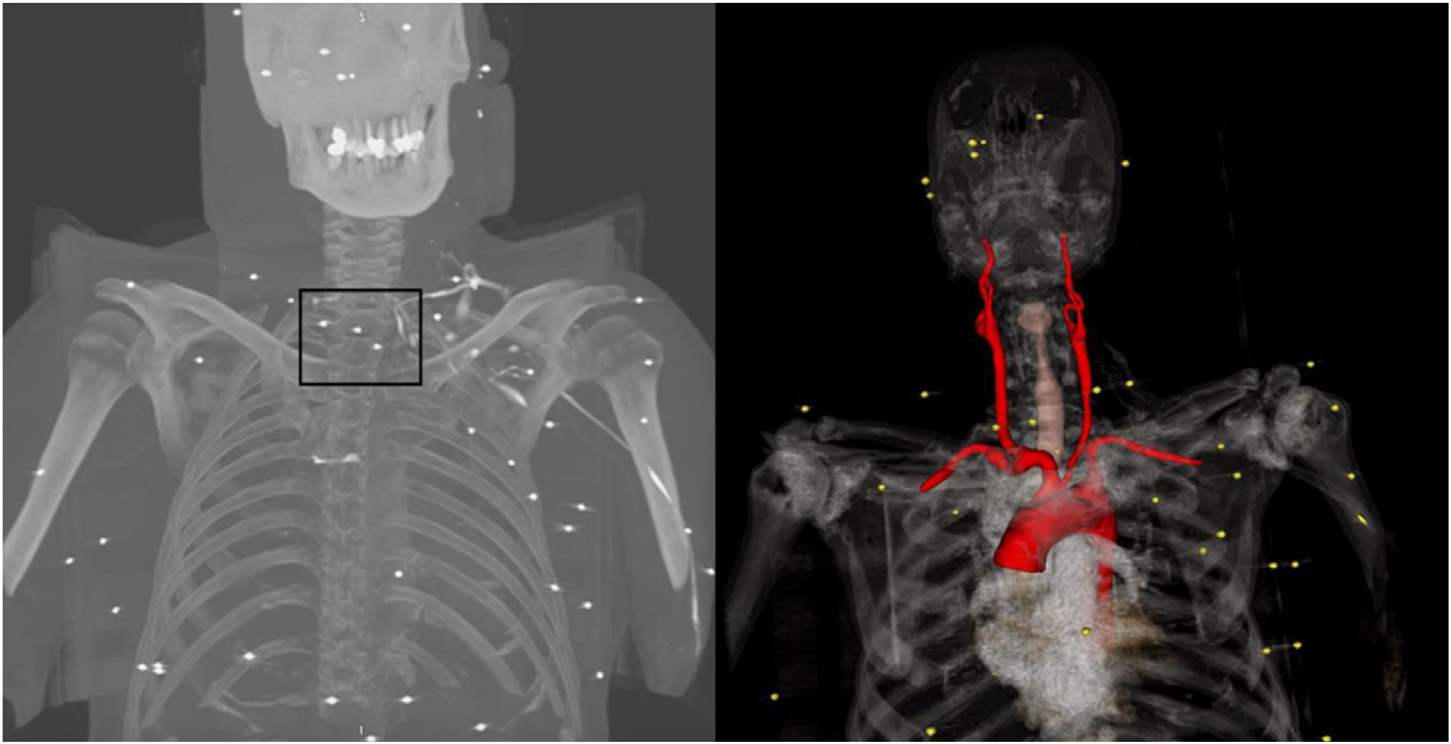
Computed tomography angiography (CTA) image demonstrating multiple metallic pellets distributed across the neck, thorax, and upper limbs.
